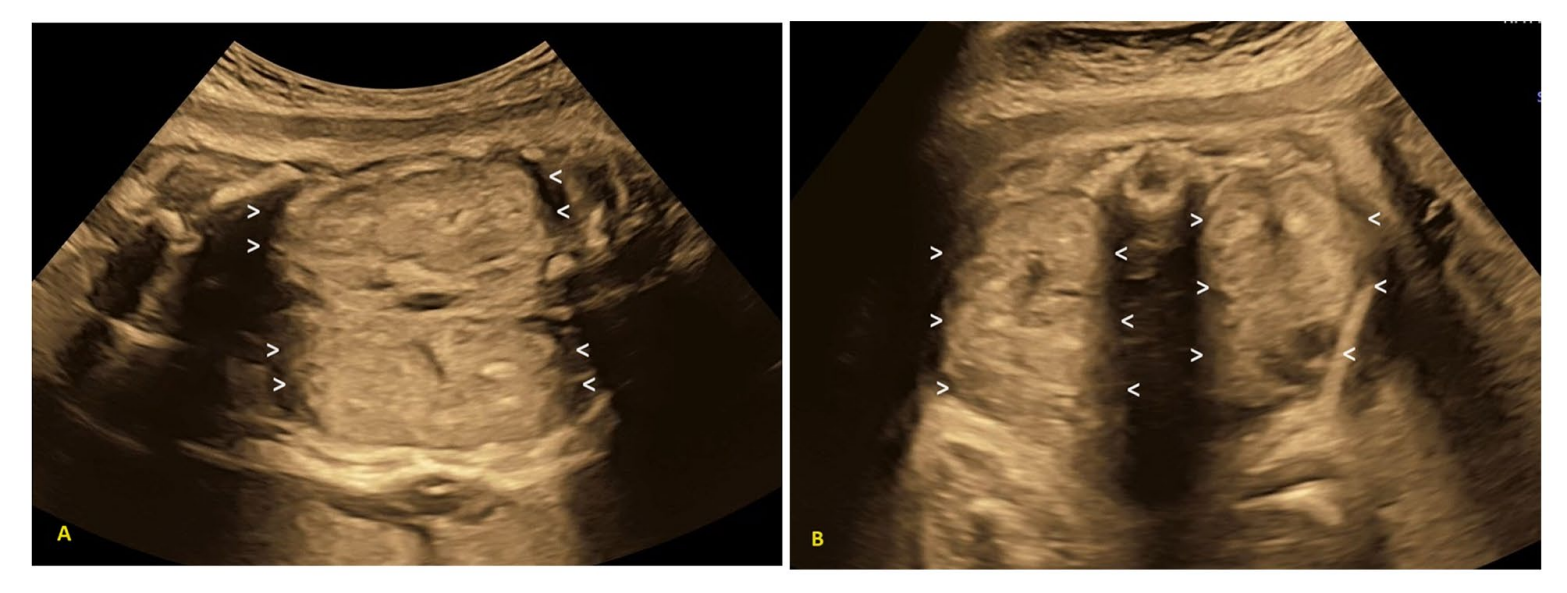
2D grey scale ultrasound of the bilateral enlarged echogenic fetal kidneys with loss of corticomedullary differentiation. The absence of fluid surrounding the fetus was also noted. A Coronal view; B Axial view
